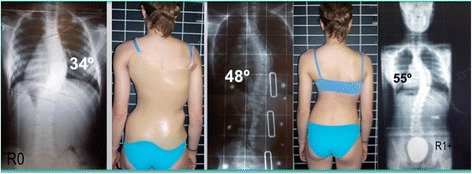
This adolescent was first seen with a right thoracic curve measuring 35°. She was recommend wearing a Chêneau-type brace and received a totally wrong designed brace (deficient four-curve design for her three-curve pattern). The X-ray in brace showed an increased angle of 48° with a change in the curve pattern, adding on the curve some lumbar vertebrae due to the inexplicably strong left thoracolumbar pad. The girl was recommended to continue wearing the wrong brace with no modifications. One year later, a new X-ray out-brace showed a curve progression to 55° Cobb. The persistence of the original curve pattern, demonstrated the improper action of the left thoracolumbar pad, real cause of a temporary in-brace adding on phenomenon
